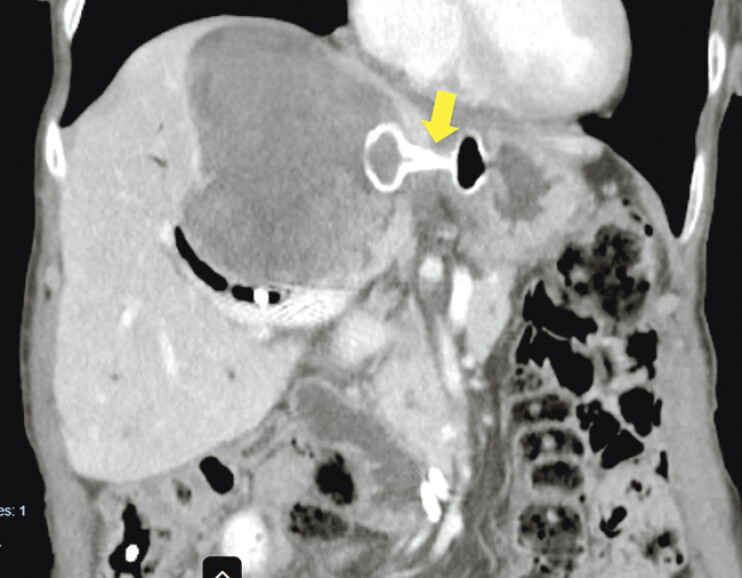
Computed tomography (CT) scan showing a correctly placed 20-mm lumen-apposing metal stent (arrow) connecting the intrahepatic cyst and the stomach.
